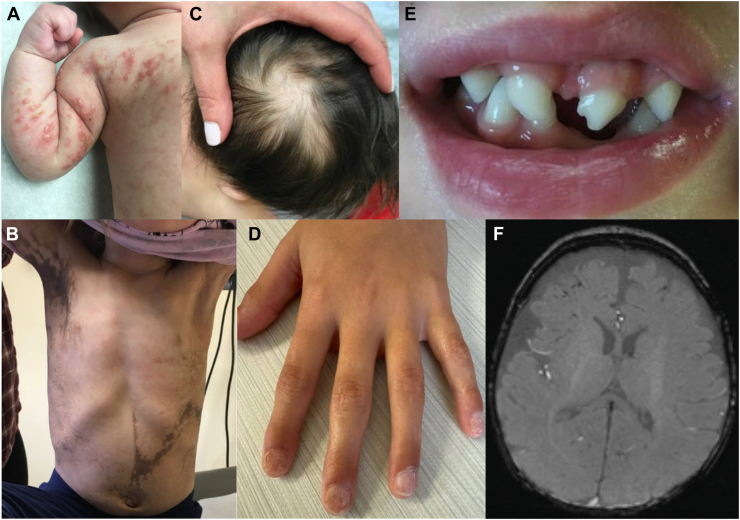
Systemic findings of incontinentia pigmenti. Skin findings include blistering (A) and hyperpigmentation (B). Other findings include alopecia (C), dystrophic nails (D), and abnormally shaped teeth (E). Magnetic resonance imaging of the brain (F) shows hyperintense lesions in the bilateral frontal subcortical region, which is concerning for subacute to old microhemorrhages that can be associated with incontinentia pigmenti.
